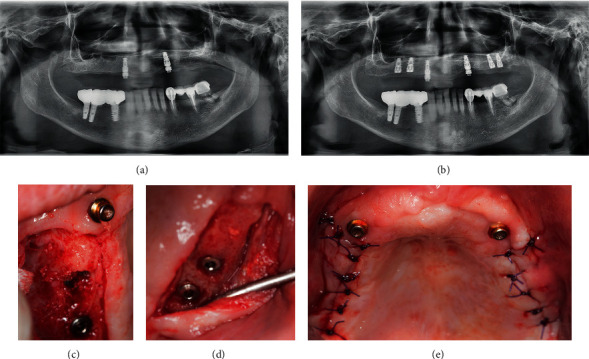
Following sinus graft maturation: (a) Panoramic x-ray 6 months following segmental osteotomy along bilateral sinus augmentation. (b) Panoramic x-ray, 8-months following segmental osteotomy along with bilateral sinus augmentation, and 2 months after implant placement in the sinuses region. (c, d) Two implant placement per each sinus 6-months following bilateral sinus floor elevation. (e) Occlusal view of the maxilla immediately after implant placement and flaps suturing.
